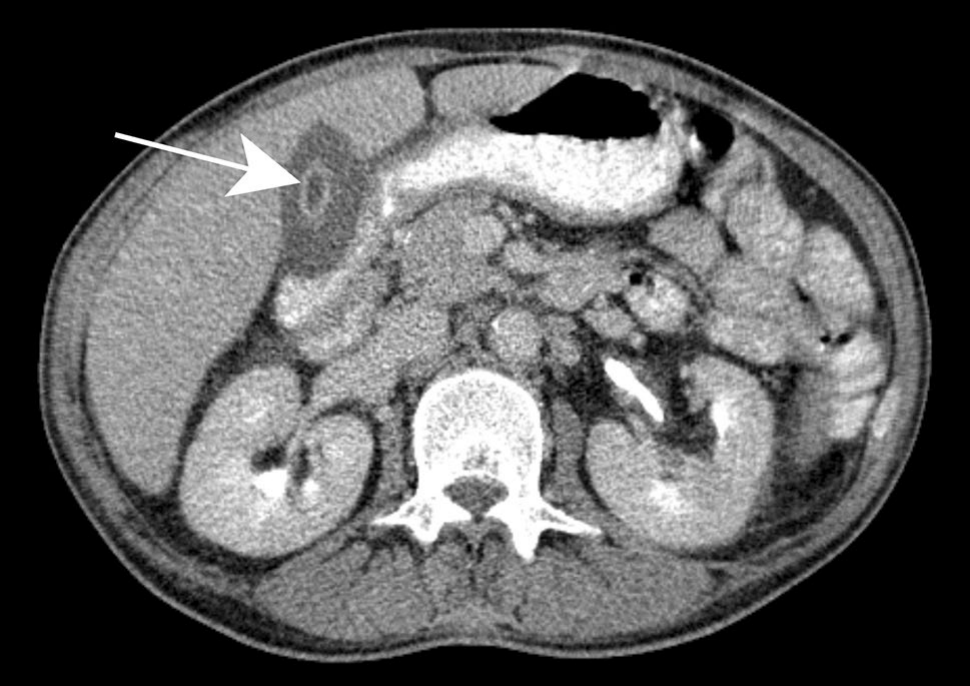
Acute viral hepatitis in a 59-year-old male patient with jaundice and elevated liver enzymes due to hepatitis A infection. Axial contrast-enhanced CT shows a contracted gallbladder with a thick hypoattenuating edematous wall and an enhancing mucosal layer (white arrow)
